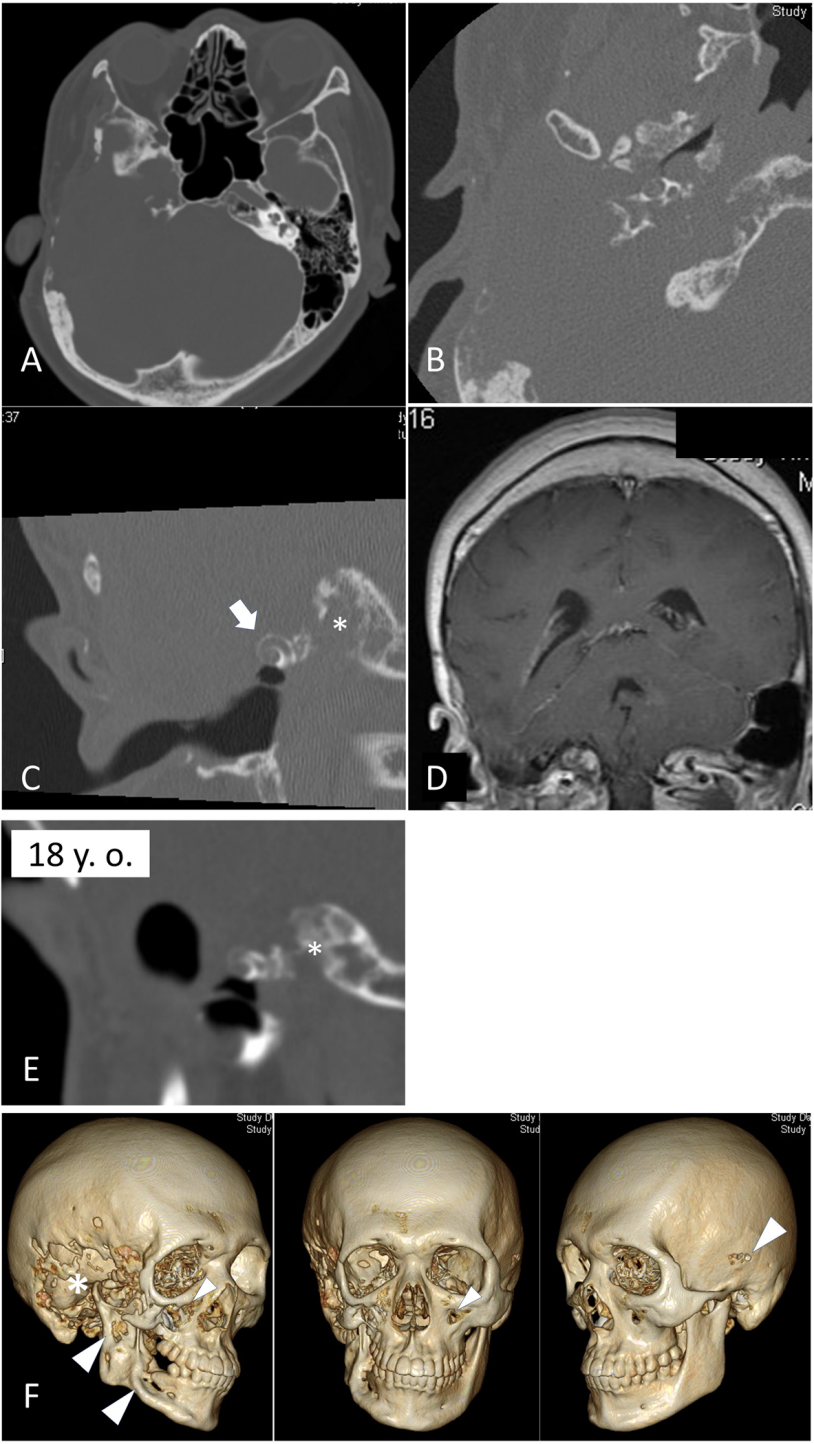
CT scans and MRI showing massive osteolysis of the skull bones. a−c The CT scans show that despite osteolysis affecting most of the temporal bone, the cochlear bony capsule was preserved and is almost intact (arrow in c). MRI revealed that the temporal lobe of the brain had herniated into the melted mastoid (d). The CT scans reveals progressive osteolysis of the temporal bone (asterisk in c and e) comparing with previous imaging obtained when he was 18 years old (e). 3D reconstructed CT clearly shows osteolysis of the skull bones; in addition to the right temporal bone (*), other affected bones include the mandibular bone, zygomatic bone, and contralateral temporal bone (arrowheads in f)
